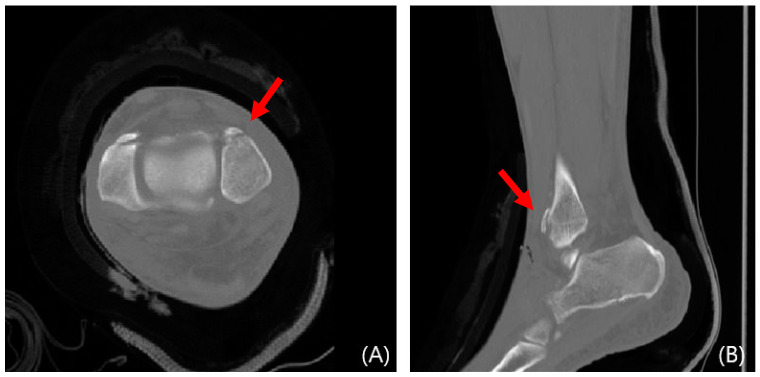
Preoperative CT images of a 47-year-old female with a Wagstaffe fracture (red arrow). Axial (A) and sagittal (B) views.
